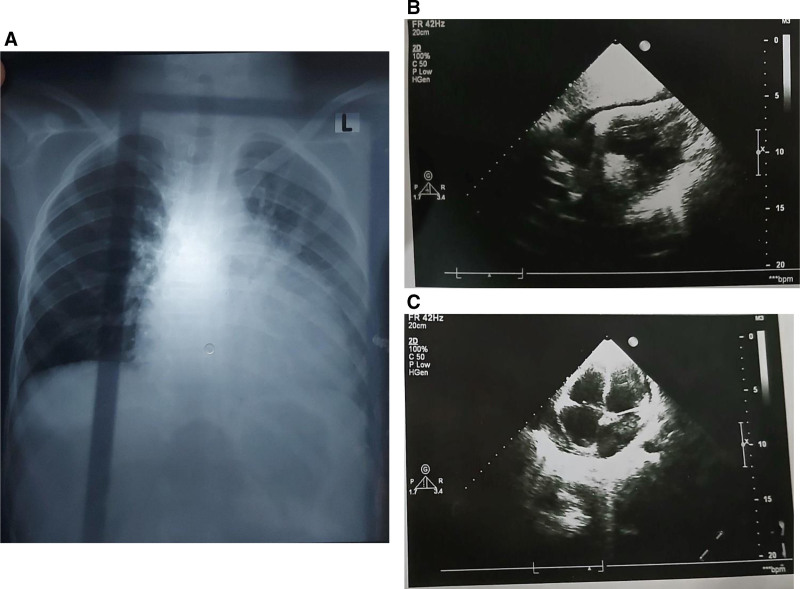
Radiographic evaluations of the patient for serositis. (A) Chest radiograph demonstrating an enlarged cardiac silhouette with clear lung fields and no pleural effusion. (B) and (C) Parasternal long- and short-axis transthoracic echocardiographic views, respectively, showing balanced cardiac chambers and a thin, circumferential pericardial effusion, with preserved systolic function.
